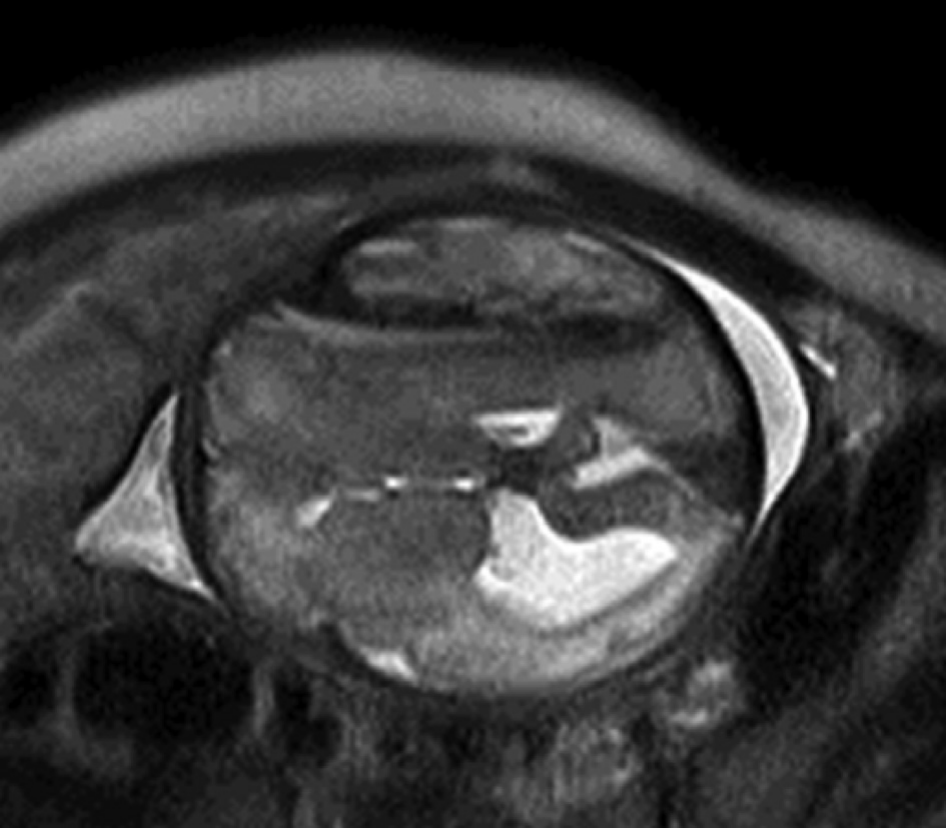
Head of the fetus in the mother's pelvis. The T2-weighted MRI images show hematoma in the bilateral subdural areas and enlargement of the left lateral ventricle due to compression.
